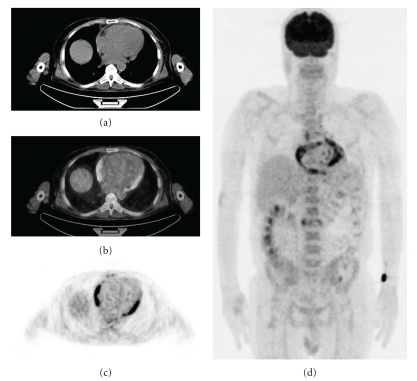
PET-CT of a 63-year-old man with FUO. Transaxial slices of CT (a), fusion (b), PET (c), and anterior MIP image (d) demonstrated increased uptake of FDG in the pericardium. Viral pericarditis was diagnosed by fine needle aspiration biopsy.
